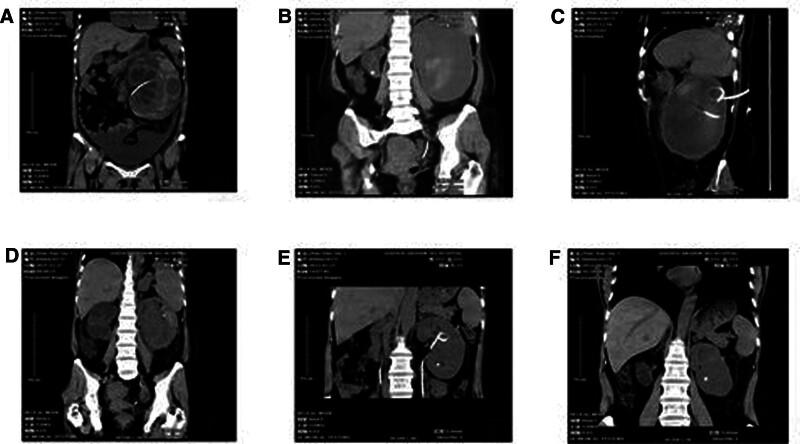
CT images of the patient during treatment. (A) The left ureteral stent was placed. (B) After the patient returned to our hospital, our reexamination of the CT scan showed that the perirenal hematoma had worsened compared to before and there was evidence of urinary extravasation. (C) The reexamination showed the situation of 2 drainage tubes. (D) Following placement of the nephrostomy tube, the patient had a fever and a reexamination CT showed hydrops in the right kidney. (E) The CT scan showed a significant reduction to hematoma and urine extravasation before removing Bilateral ureteral stents. CT = computed tomography.
